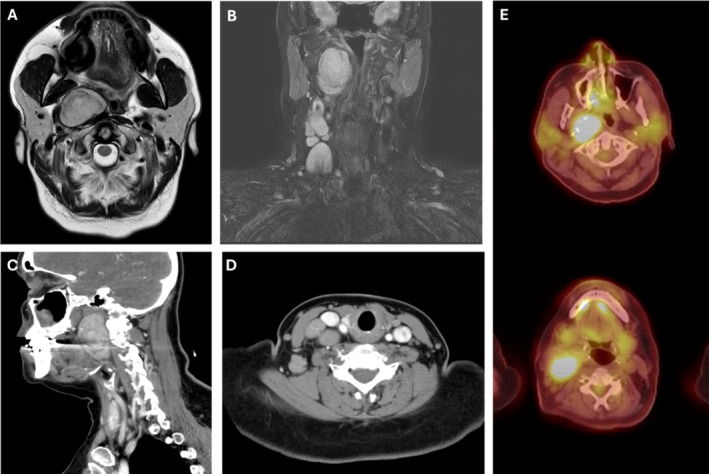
(A) Initial axial and (B) coronal T2 weighted MRI revealing a 4.0 cm mass in the right parapharyngeal space with ipsilateral lymphadenopathy. (C) Subsequent sagittal and (D) axial CT with contrast showing interval growth of the paraphyangeal space mass to 5.0 cm and stable lymph nodes. (E) Axial PET scan with FDG‐avid right‐sided parapharyngeal mass and lymph nodes. [Color figure can be viewed in the online issue, which is available at www.laryngoscope.com]
